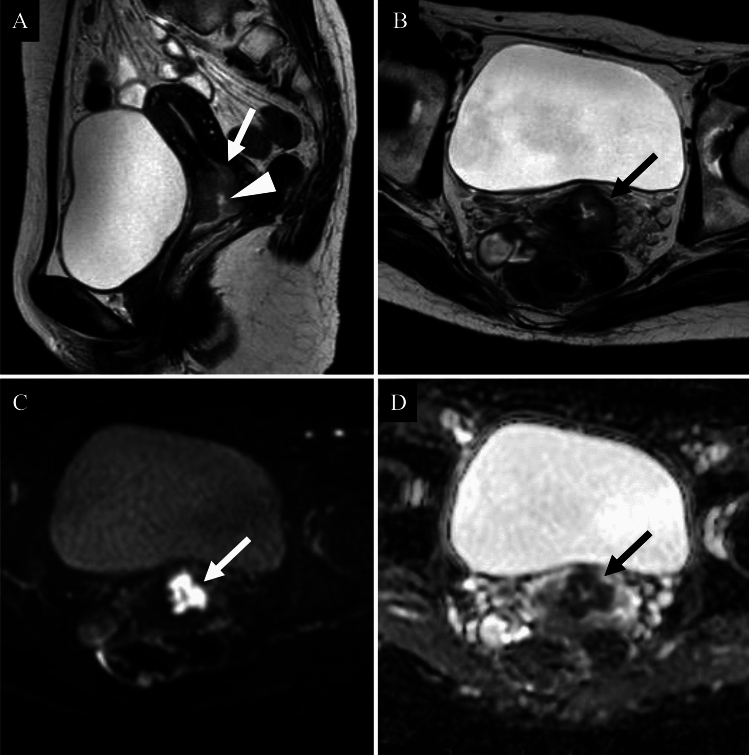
Squamous cell carcinoma of the uterine cervix in a woman in her fifties. A Sagittal T2-weighted image; B Axial T2-weighted image; C Axial diffusion-weighted image; D Axial apparent diffusion coefficient map. A cervical mass exhibiting high signal intensity relative to the surrounding stroma on T2-weighted image is identified (A, B: arrows). A central depression consistent with ulceration is observed (A: arrowhead). The lesion shows a markedly high signal on diffusion-weighted image (C: arrow) and significantly reduced apparent diffusion coefficient values (D: arrow), with a mean ADC of 0.783 × 10⁻^3^ mm^2^/s. The tumor is confined to the uterus, with no evidence of parametrial invasion. A diagnosis of FIGO 2018 stage IIA1 HPV-associated squamous cell carcinoma was confirmed following surgery
